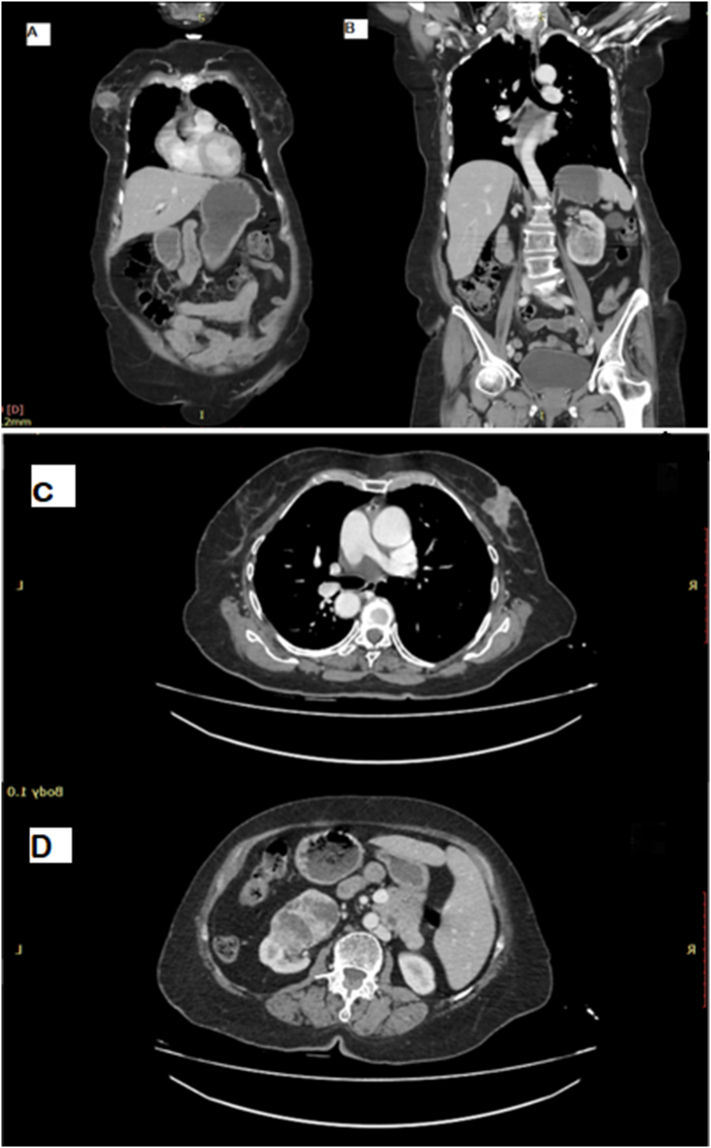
CT Chest abdomen and pelvis (axial views) showing right breast Ca (A) and left renal Ca (B) coronal views showing right breast Ca (C) and left renal Ca (D).
